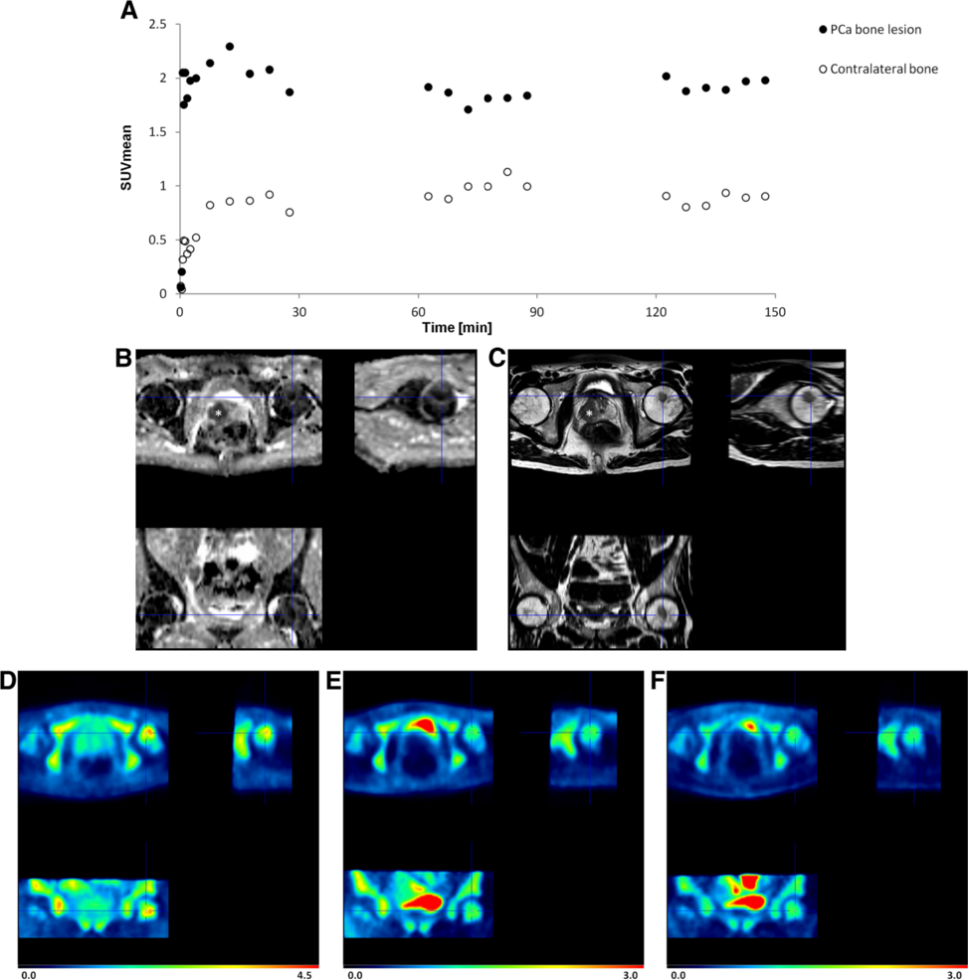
Kinetics of ^18^F-MK-9470 in a bone metastasis. ^18^F-MK-9470 uptake in a lesion of the left femoral head versus ^18^F-MK-9470 uptake in contralateral normal bone tissue of patient 5 (A). The DWI MRI (B) and the T_2_w MRI (C) images show a low-signal-intensity mass in the lesion of the left femoral head as well as the local tumour (indicated with an asterisk). Integrated PET images of the first (D), second (E) and third (F) acquisition time interval demonstrate increased ^18^F-MK-9470 uptake in the bone metastasis as compared to that of the contralateral bone. Note the absence of ^18^F-MK-9470 uptake in the local PCa visible on the MRI images. Data are scaled from 0 to 4.5 SUV in (C) and scaled form 0 to 3.0 SUV in (D) and (E).
